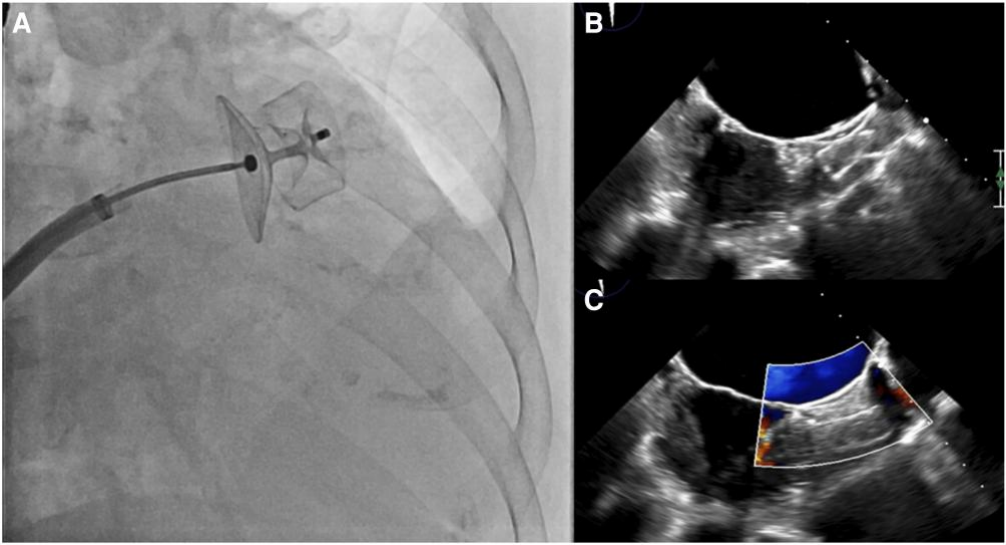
(*A*) Left atrial appendage occlusion device deployment. (*B*) Transoesophageal echocardiography post-procedure with good result without any residual leak.
